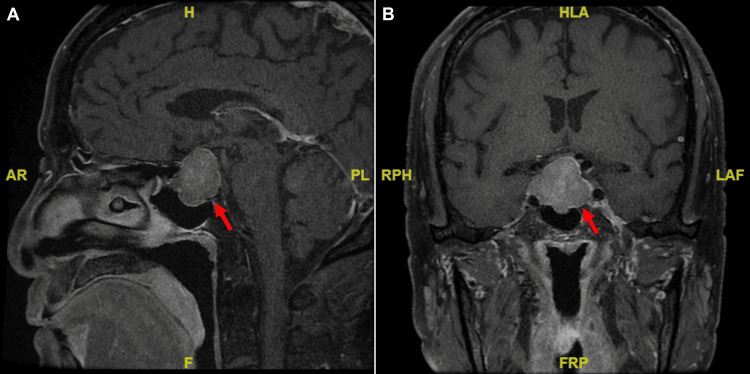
Contrast-enhanced longitudinal relaxation time (T1)-weighted magnetic resonance imaging depicts a pituitary macroadenoma (red arrows) measuring 3.1 x 2.8 x 2.2 cm, with evident compression of the optic chiasm observed in sagittal view (A) and coronal view (B).
